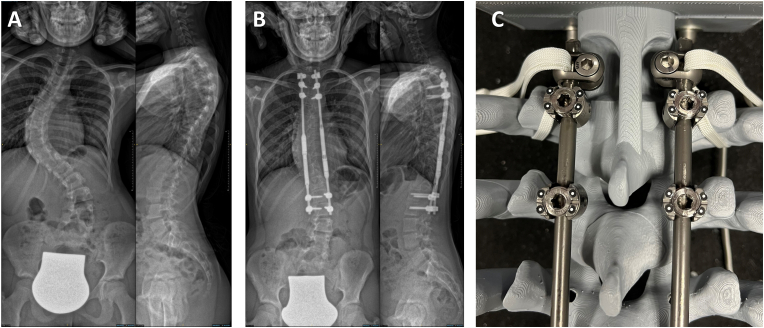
Anterior-posterior and lateral radiographs of a patient with idiopathic scoliosis (A) following MCGR implantation (B). Cranial anchoring with pedicle screws and lamina band encirclement around the second rib in a model (C).
